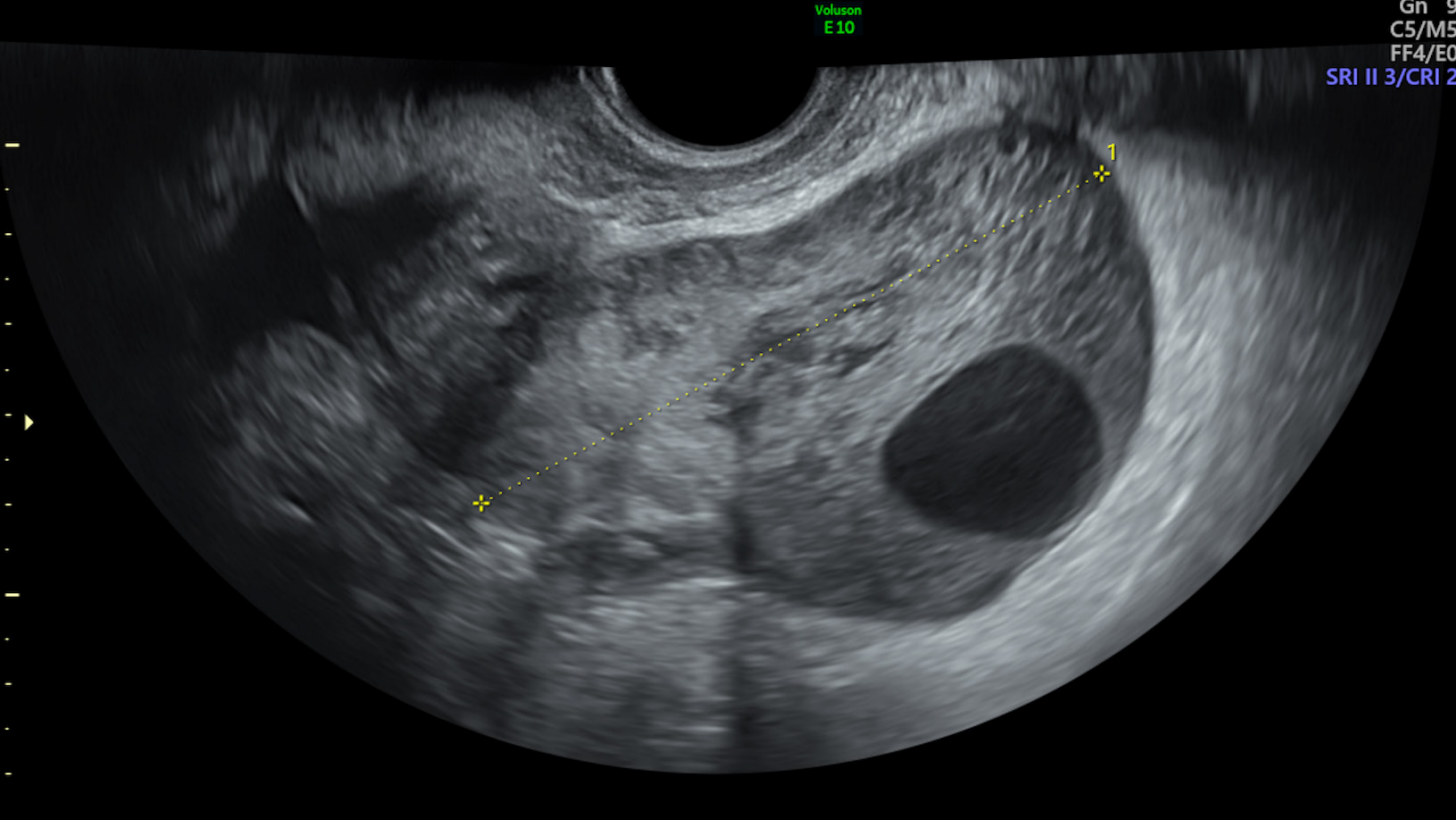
Maximum diameter at ultrasound of case 2.
